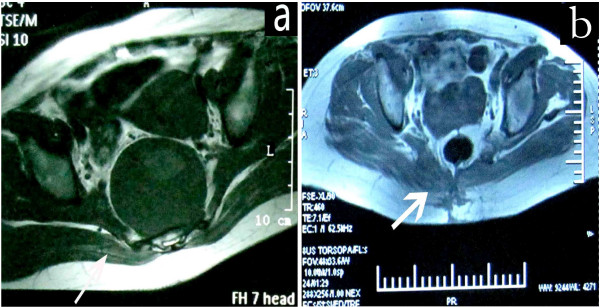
Magnetic resonance imaging of the tumor and flap. Sacral tumor and intact gluteus maximus (GLM) (arrow) before surgery (a). Well-maintained GLM adipomuscular flap (arrow) behind the rectum (b) 6 months after surgery.
